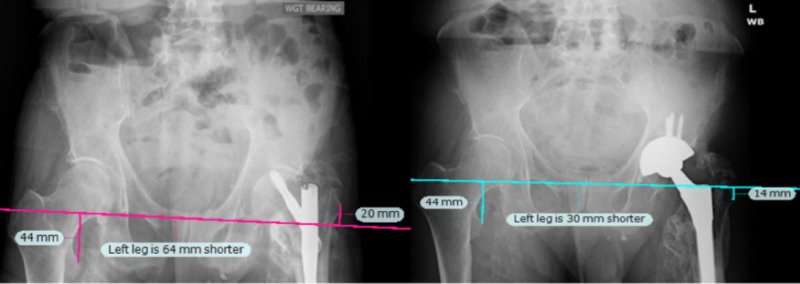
Radiographs for Case 1. Due to deformity at the operative lesser trochanter, the greater trochanter was used as a landmark for leg length calculations. Preoperatively (left), the LLD was estimated at 45 mm. The operative leg was lengthened by 35 mm during surgery, which was confirmed by both the navigation device and the post-operative radiograph (right). LLD: Leg length discrepancy
